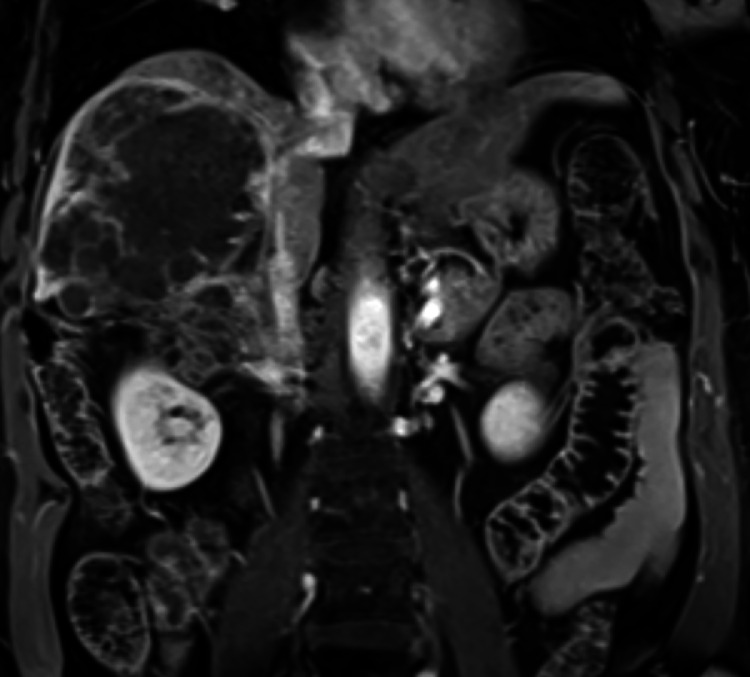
T1-weighted postcontrast MRI 4 months after radioembolization plus ipilimumab and nivolumab demonstrated positive treatment response without concerning enhancement within the right hepatic lobe HCC, now decreased in size with a maximum diameter of 11.5 cm.
